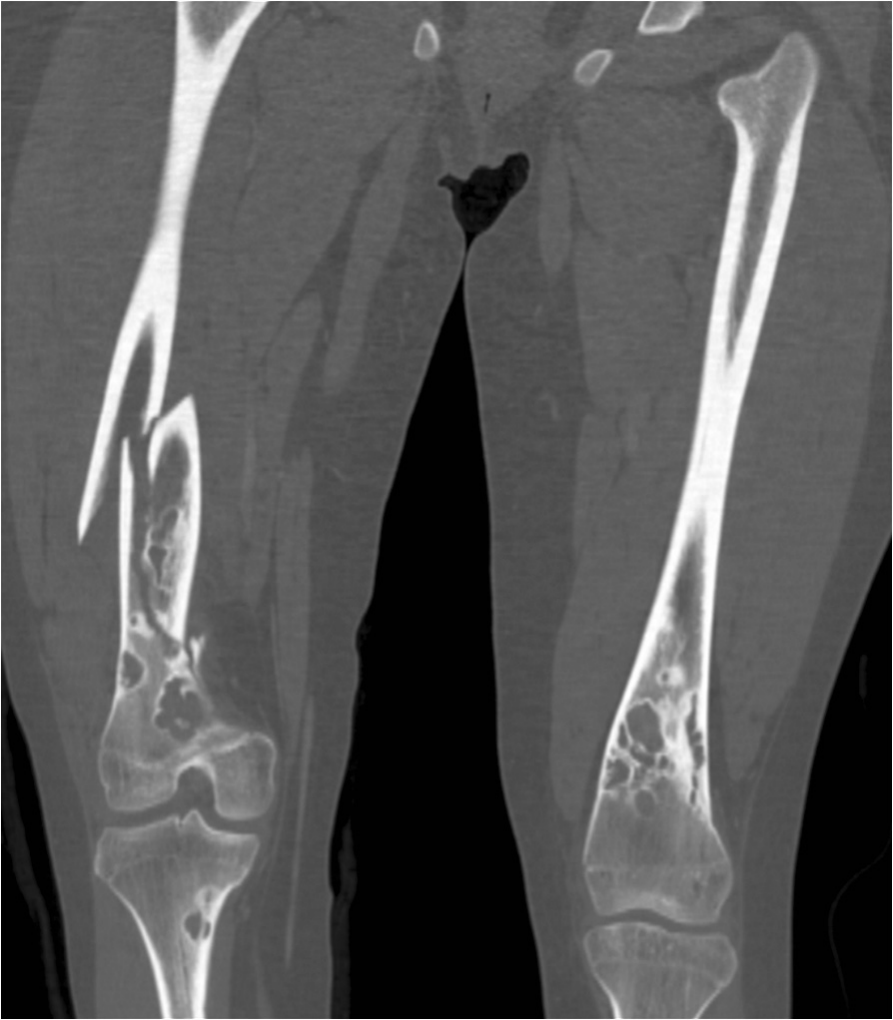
Coronal-reformatted CT image demonstrating multiple bilateral NOFs of both the distal femurs and proximal tibias.
